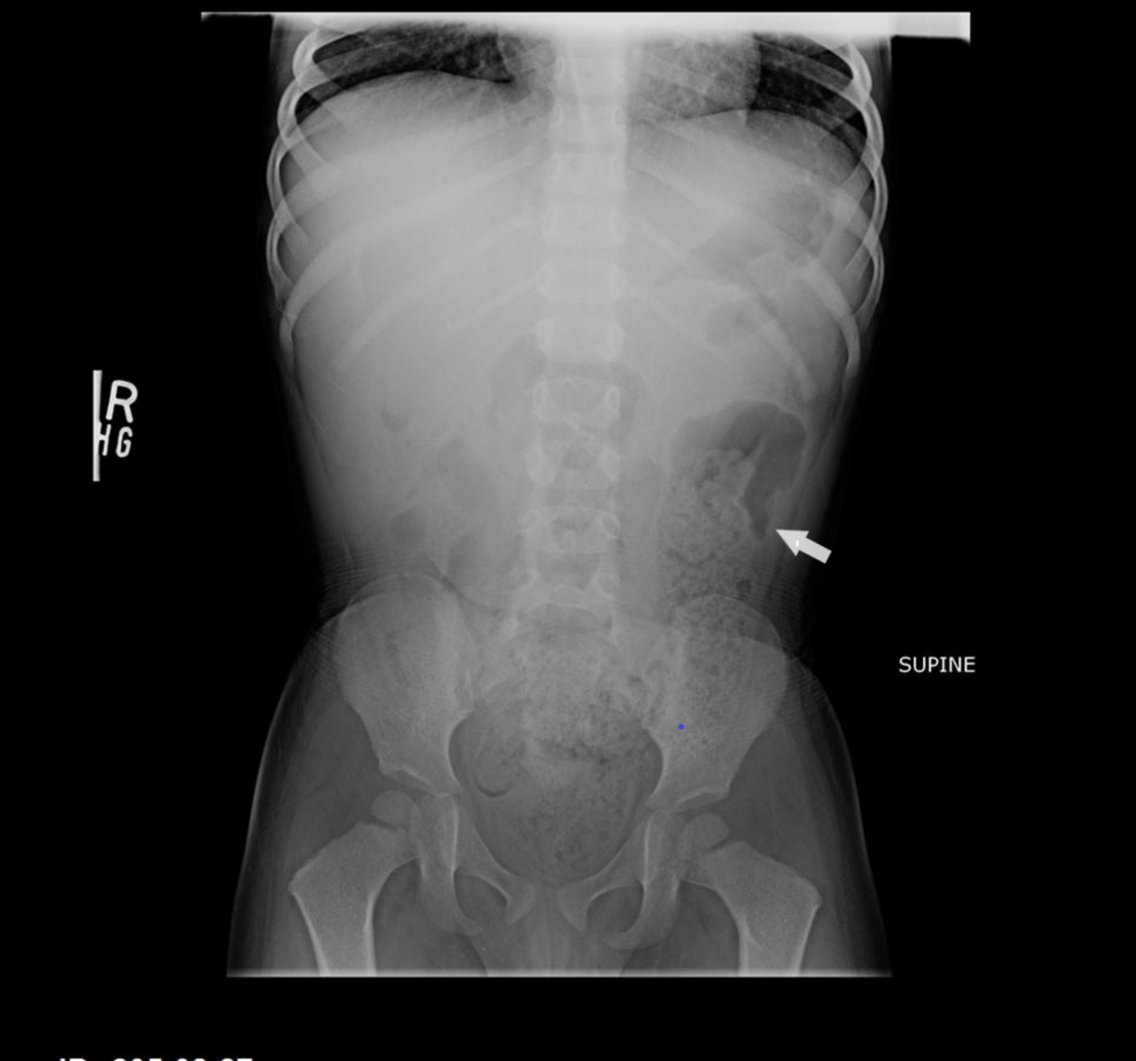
Repeat abdominal X-rays upon readmission Fecal loading seen in large bowel (arrow)
